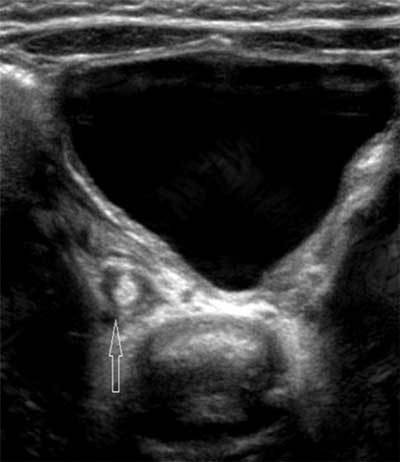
Axial ultrasound scan of the right pelvic ureter containing a fungus ball (open arrow).
